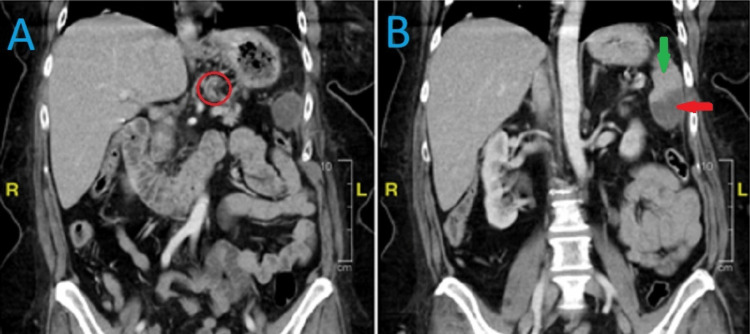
Representative image of a CT scan of the abdomen showing a filling defect indicating persistent thrombus in the splenic artery, as well as visible infarction in the spleen. (A) Area within the red circle indicates the location of the thrombus. (B) Green arrow indicates normal area of the spleen, which is hyperdense. Red arrow indicates the infarcted area of the spleen, which is hypodense.
